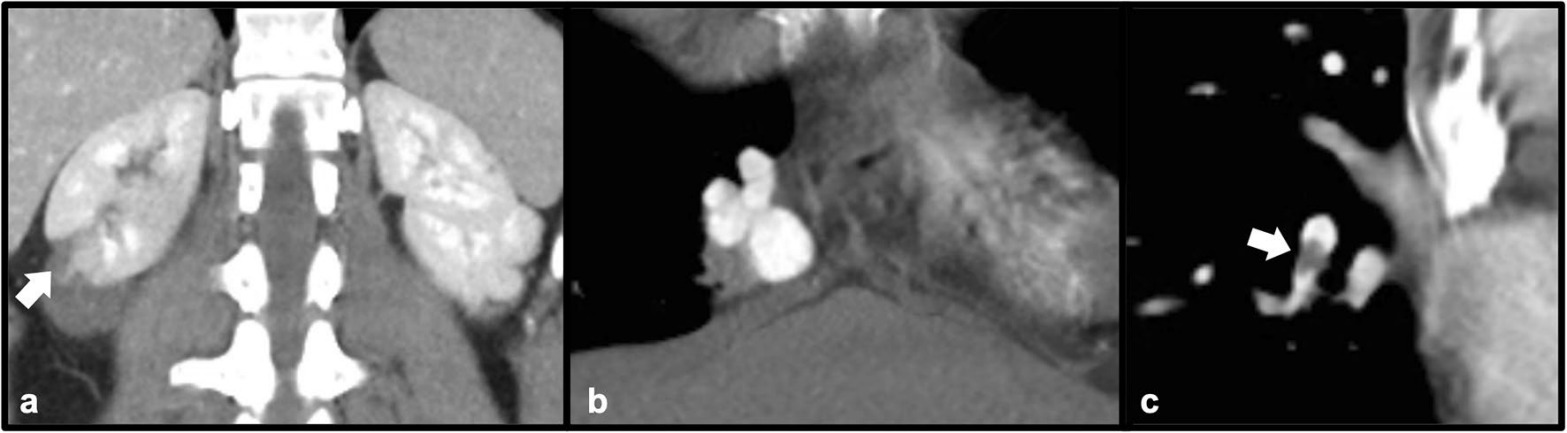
42-year-old female with right lower quadrant abdominal pain. Coronal contrast-enhanced CT (a) demonstrates a wedge-shaped cortical defect in the right inferior renal pole (white arrow) consistent with renal infarct. Coronal contrast-enhanced CT pulmonary angiogram (b and c) demonstrates an enlarged, tortuous pulmonary artery with pulmonary venous communication consistent with pulmonary AVM. A filling defect (white arrow) can be seen within a pulmonary artery supplying the AVM consistent with pulmonary embolism, with probable embolic fragments passing through the AVM due to right-to-left shunting, leading to embolic renal infarction
